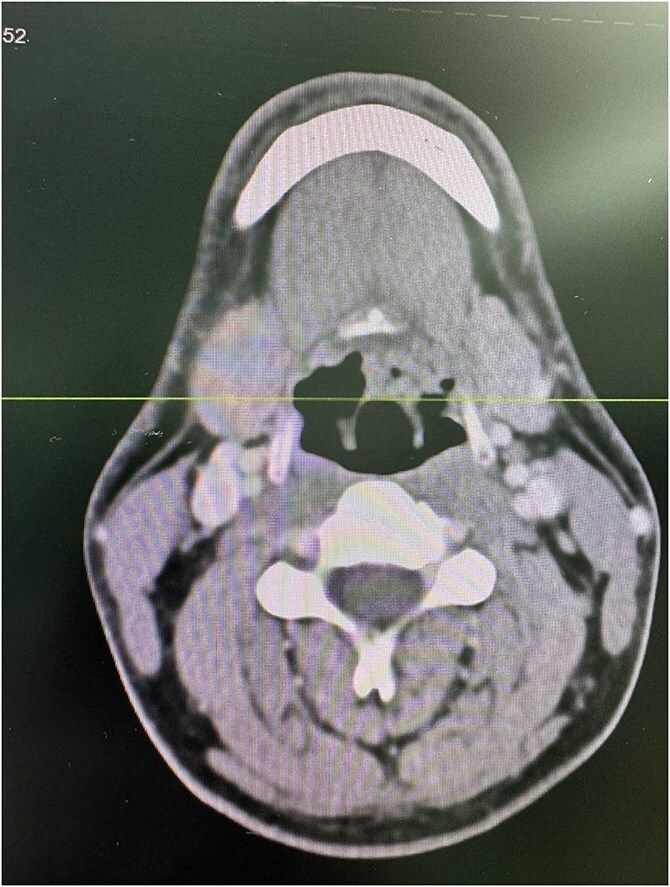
Axial CT scan of the neck with intravenous contrast demonstrating a 4 × 4 mm non-enhancing nodule (arrow) in the posterior aspect of the upper end of the left aryepiglottic fold. Asymmetry of the vallecula is noted with shallowness on the left side, likely due to the extension of the lingual tonsils.
